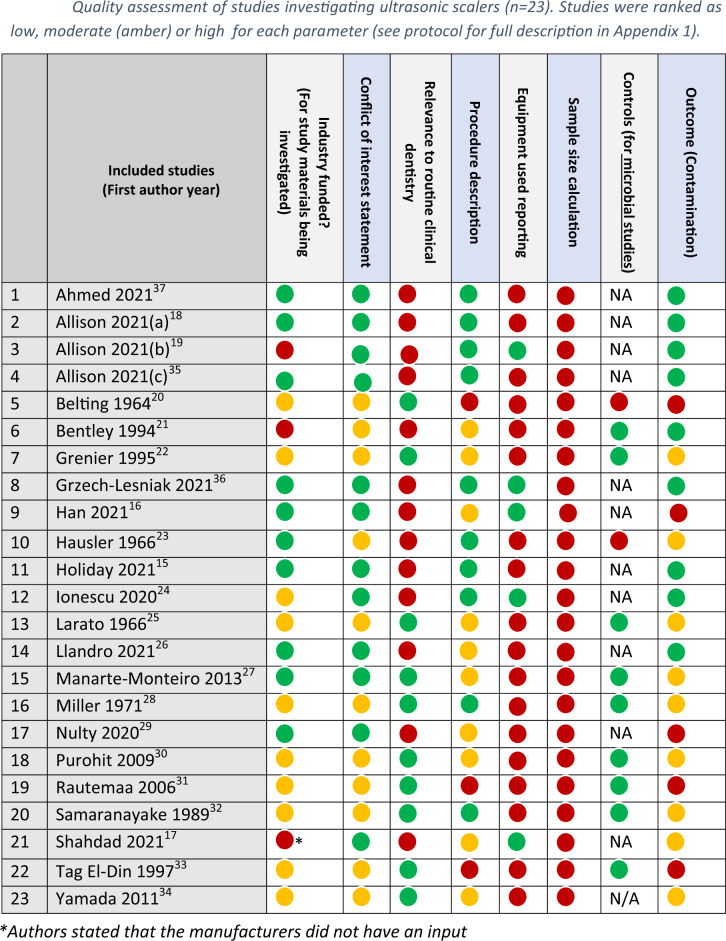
Quality assessment of studies investigating ultrasonic scalers (*n* = 23). Studies were ranked as low, moderate (amber) or high for each parameter (see protocol for full description in Appendix 1).
